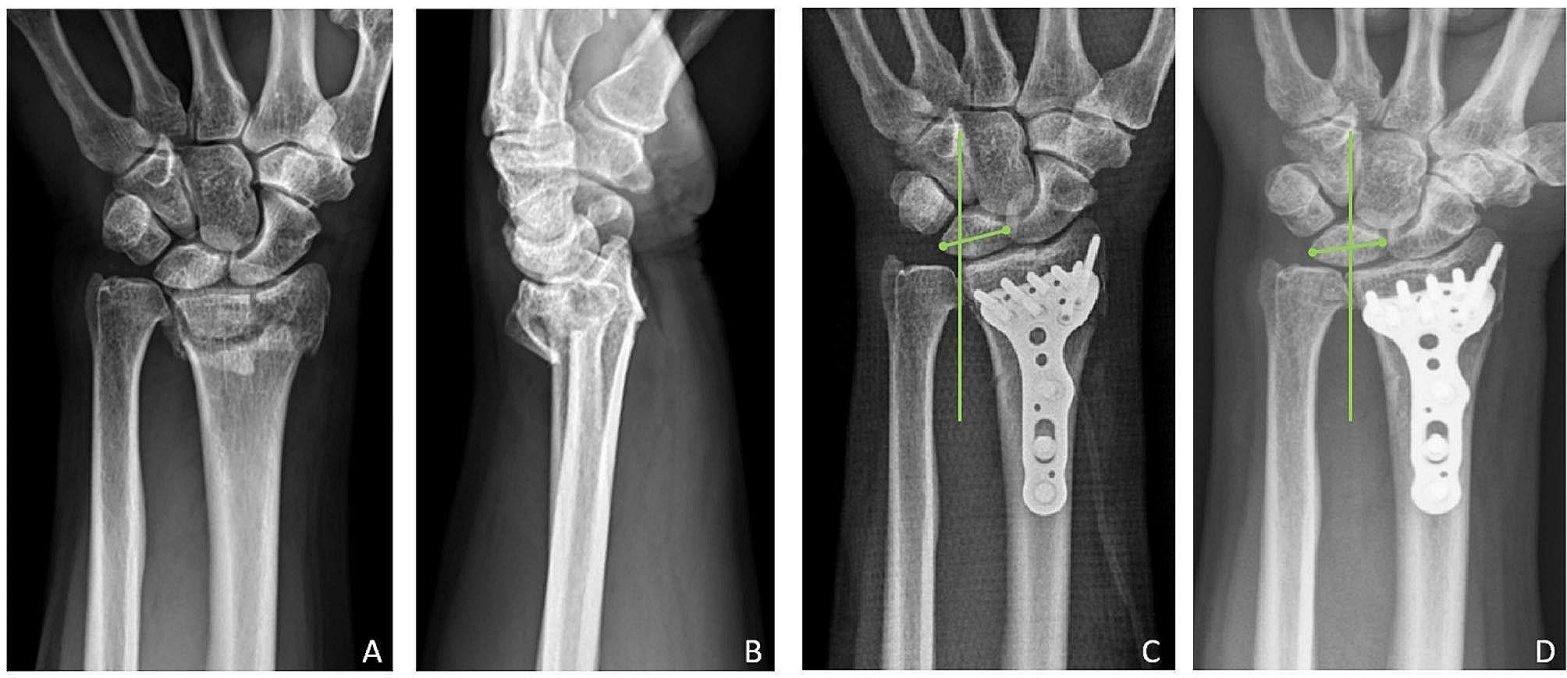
A 64-year-old man with left distal radius fracture. Radiographs showing (A) and (B) the fracture before treatment. (C) Radiograph after open reduction and internal fixation. (D) Ulnar carpal translation (UCT) was observed in the 3-month postoperative radiographs
